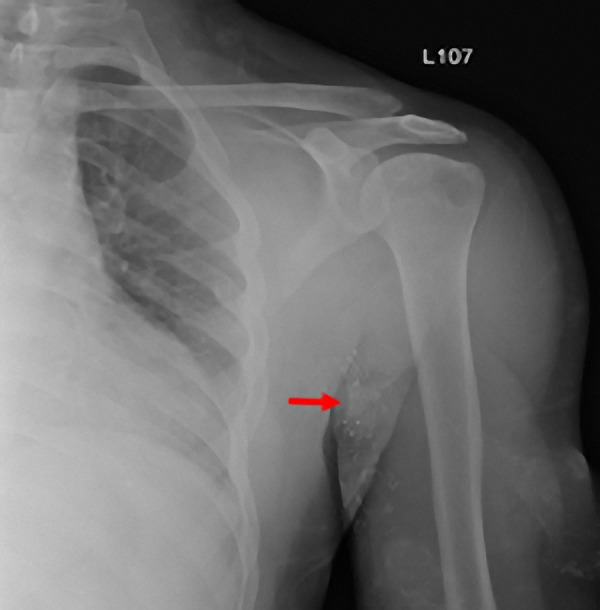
Incomplete venous stent in the left upper extremity as shown by X-ray (red arrow).
